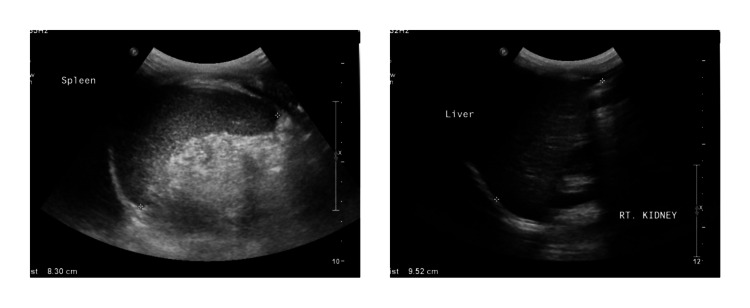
Ultrasound scan shows mild enlargement of the liver (right) and spleen (left).
